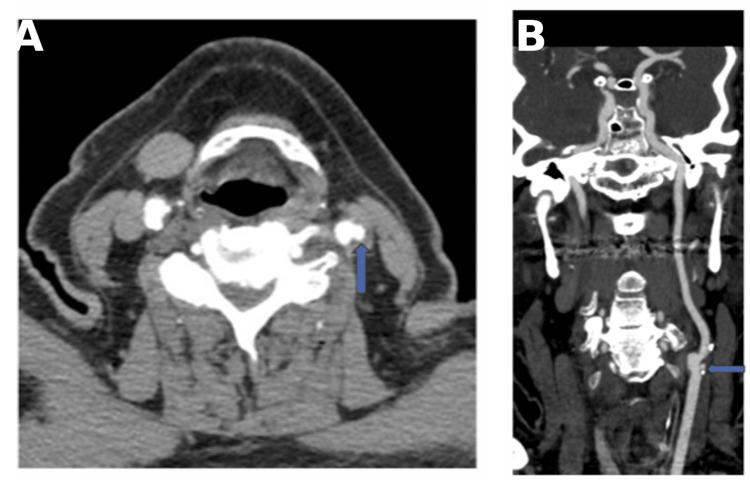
Case 1: A (axial) and B (coronal) reconstruction CTA revealing a soft plaque at the left carotid bifurcation with a 5 mm plaque ulceration (arrows) and approximately 40% stenosis by the NASCET criteria. CTA: CT angiogram; NASCET: North American Symptomatic Carotid Endarterectomy Trial
